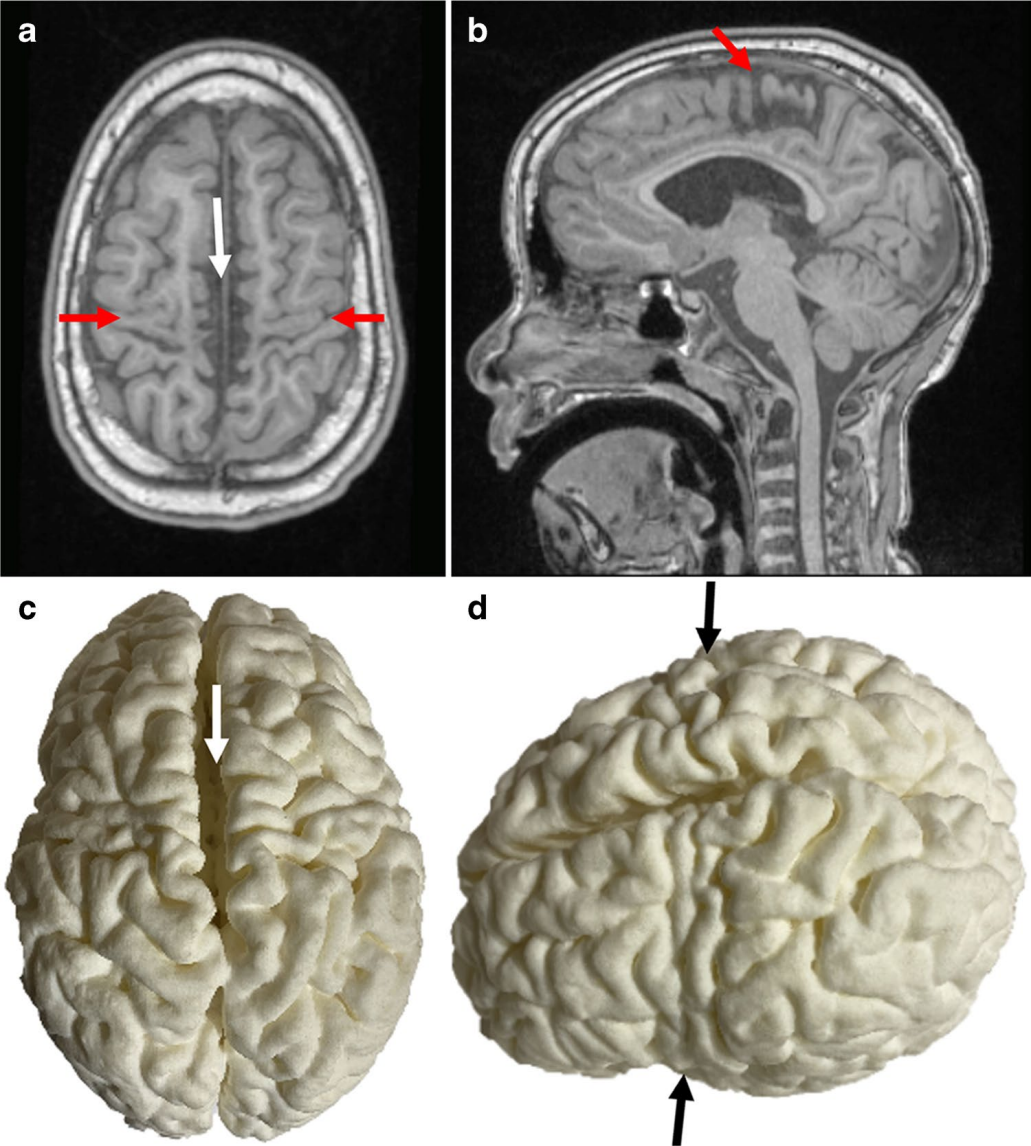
Images of an 11-year-6-month-old girl presenting with cerebral palsy. a, b Axial (a) and sagittal (b) T1-weighted magnetic resonance images show localized perirolandic atrophy and signal change (*red arrows*) and lentiform separation of the cerebral hemisphere (*white arrow*). c Vertex (c, d) and lateral oblique (d) 3-dimensional printed models with accurate depiction of the lentiform separation (*white arrow*) and perirolandic atrophy (*black arrows*)
